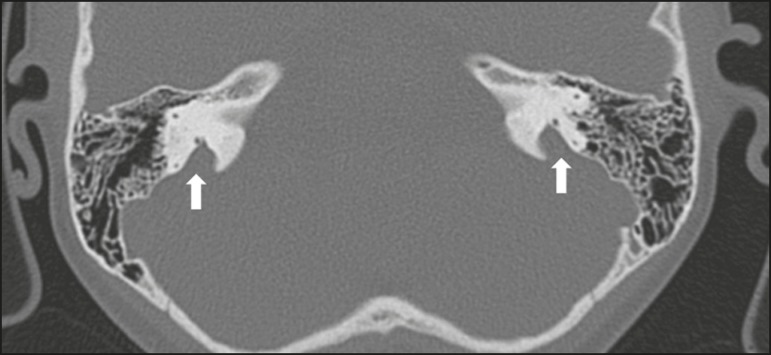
Enlarged vestibular aqueducts. Axial CT scan, with bone window
